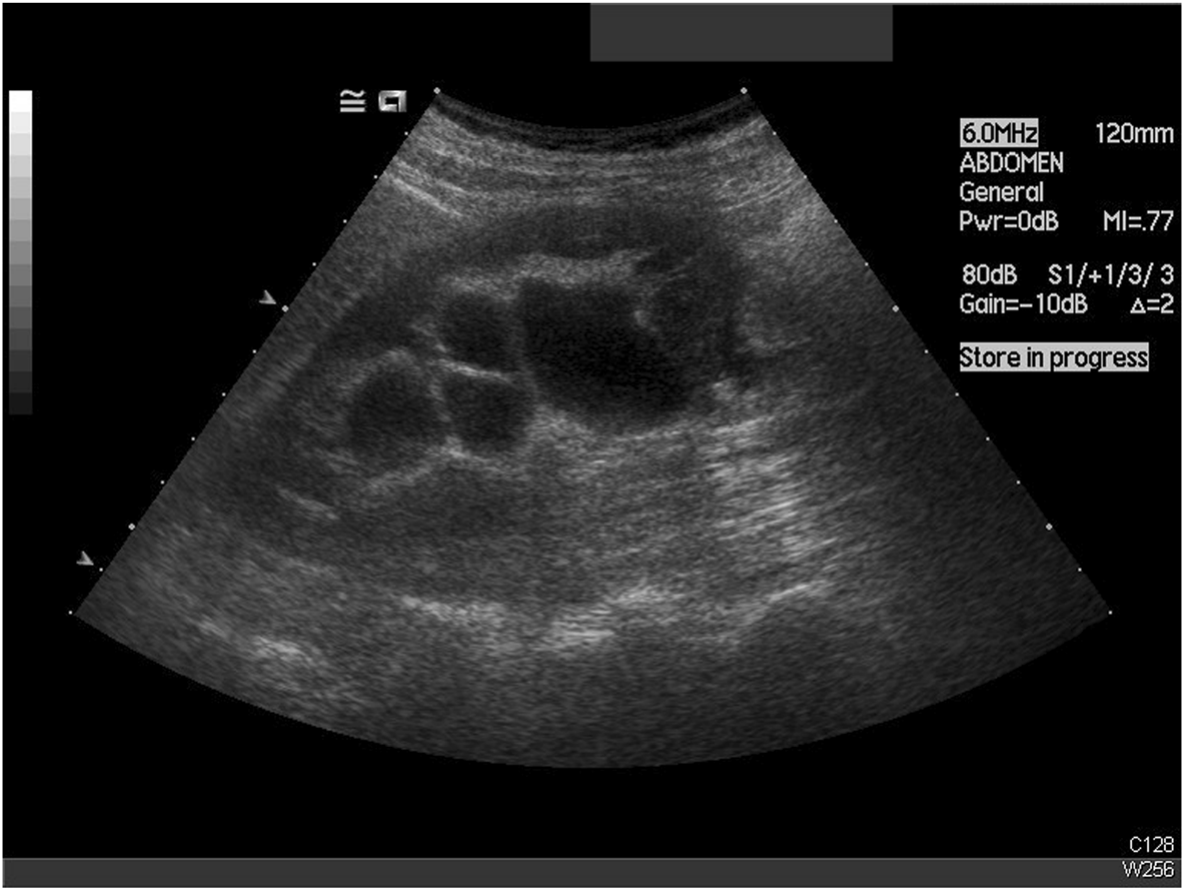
Ultrasound demonstrating hydronephrosis.
